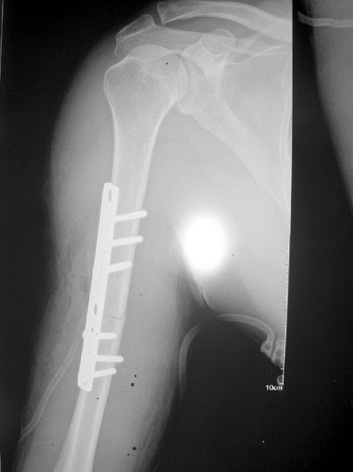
Radiographie d’une luxation antérieure de l’épaule associée à une fracture de la diaphyse humérale homolatérale
